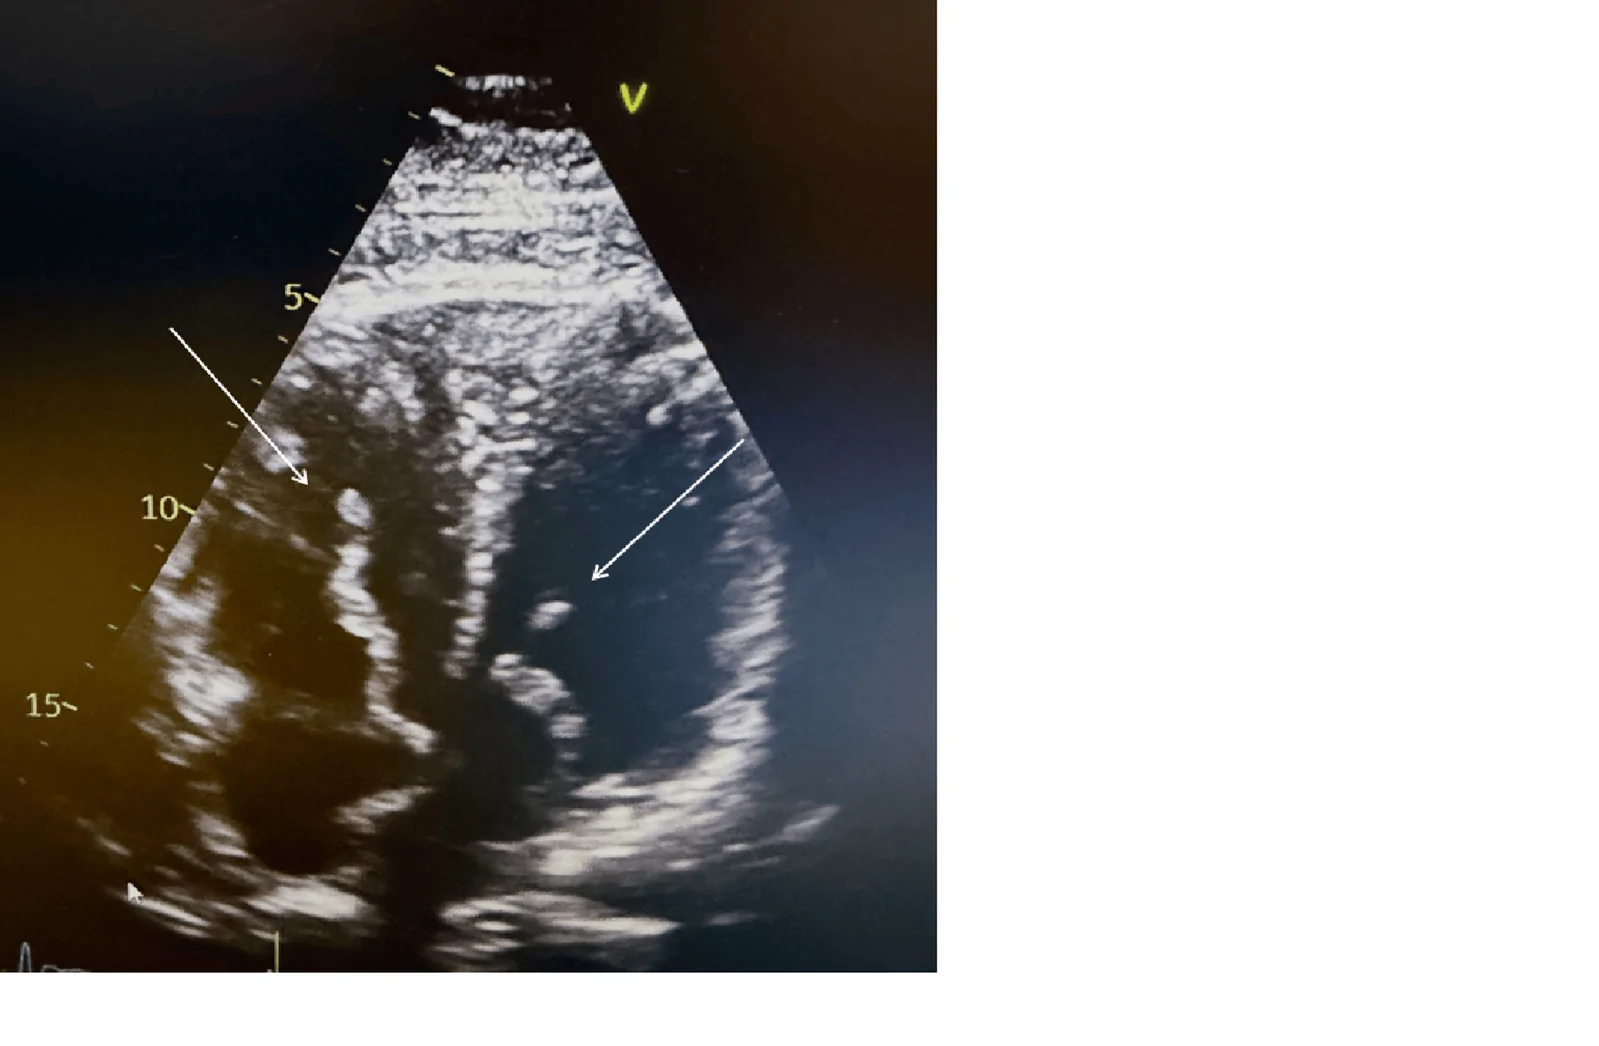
Apical four-chamber echocardiographic image demonstrating the single clot straddling the bilateral atrioventricular (AV) valves. The clot was mobile across both AV valves (arrows pointing at the free ends in each ventricle)
